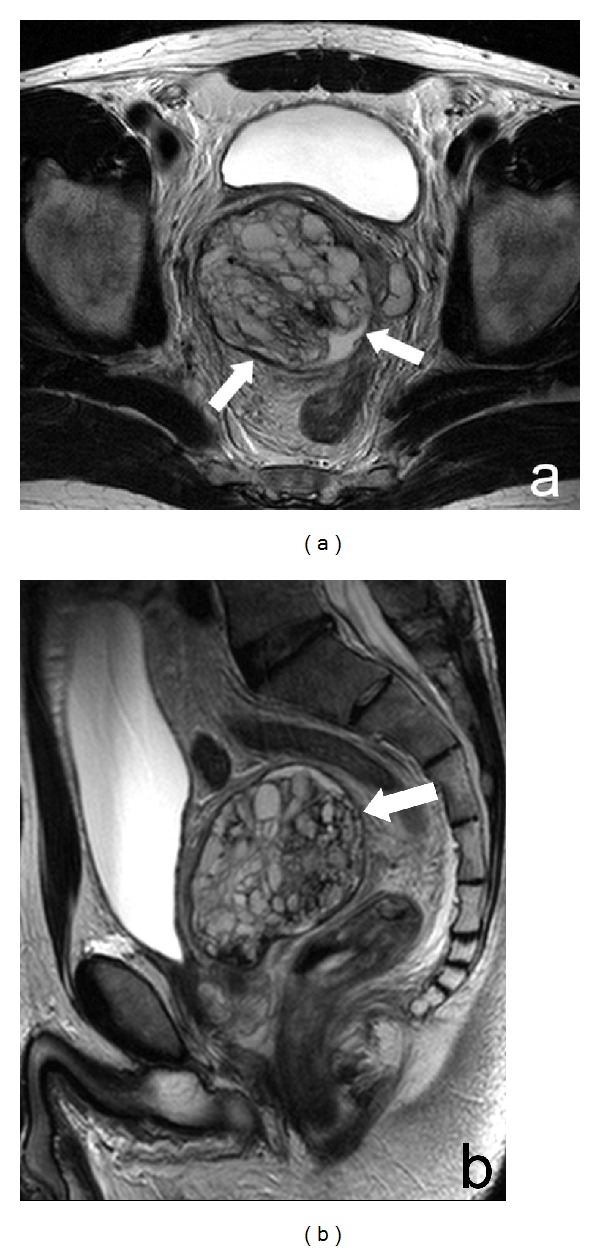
MRI of the pelvis. Axial (a) and sagittal (b) T2 sequence. Large mixed solid-fluid mass of the right seminal vesicle (arrows) without infiltration of adjacent structures.
